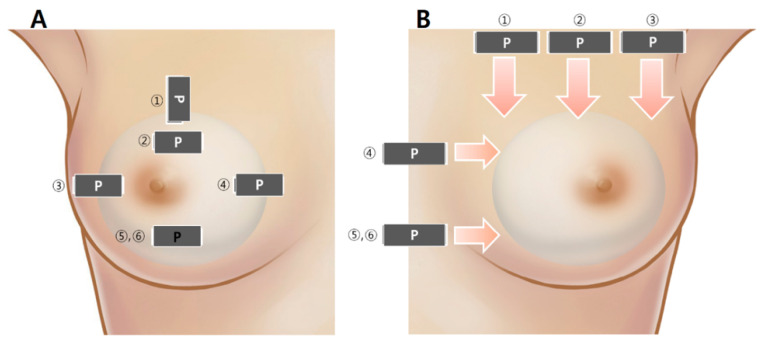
Position of breast implant ultrasonography. (A) Checkpoint for picture; (B) checkpoint for video (P is point of prove).
